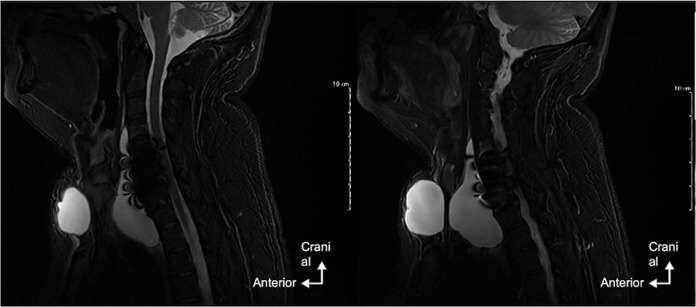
MRI in sagittal T2 section showing the anterior meningocele
